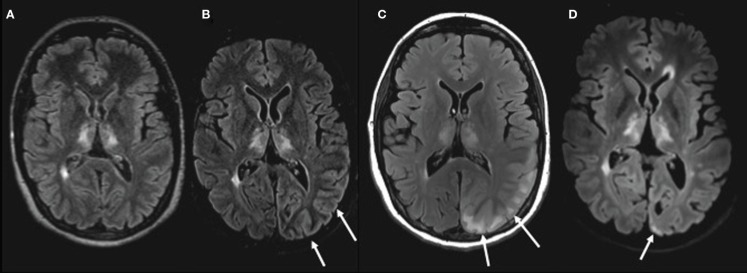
MRI of the brain performed with FLAIR sequences on four different scanners. (A) Before PRES in July 2013 (3D FLAIR 1,5T). (B) First MRI on day 4 during PRES with subtle cortical edema (arrows; 2D FLAIR 1,5T). (C) Second MRI on day 24 during PRES with cortical and subcortical vasogenic edema (arrows; 2D FLAIR 3T). (D) Follow-up MRI May 2014 with small superficial residual changes (arrow) in part of the cortex (3D FLAIR 3T). Chronic neuroinflammatory lesions are seen in the thalami and periventricular white matter.
